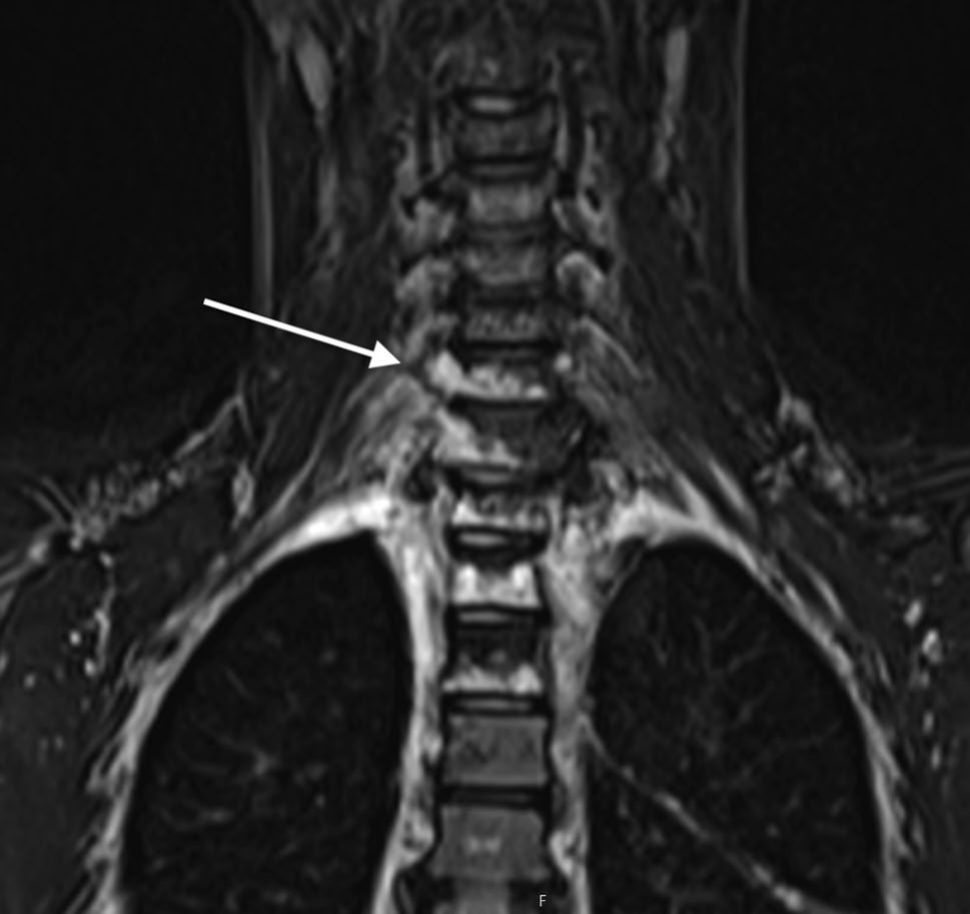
MRI showing the extent of paravertebral lymphatic malformation on the right side, also involving several vertebral bodies at the cervicothoracic junction (white arrow)
